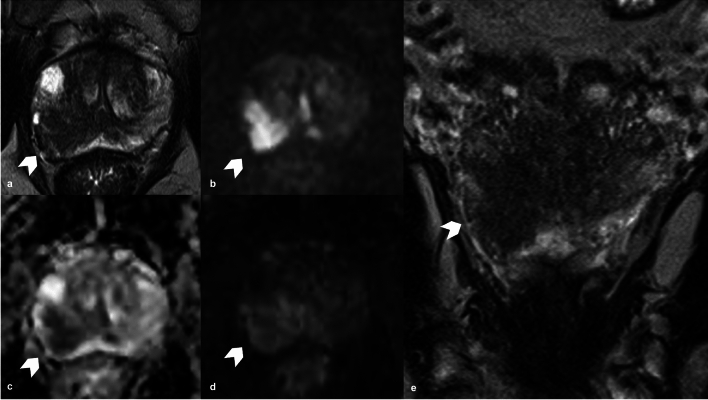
a, e T2WI (axial and coronal plane) show an alteration on the mid right posterior-lateral peripheral zone (white arrow head), distant from the NVB; b, c DWI (b = 2000) and ADC map show a focal and marked hyperintensity on high b value and hypointensity on ADC map on the right posterior lateral peripheral zone lesion (white arrow head); d DCE shows an early and focal enhancement of the lesion. All the sequences demonstrate marked capsular bulging. T2WI, T2 weighted imaging; DWI, Diffusion Weighted Imaging; ADC map, Apparent Diffusion Coefficient; NVB, neurovascular bundle; DCE, dynamic contrast enhancement
